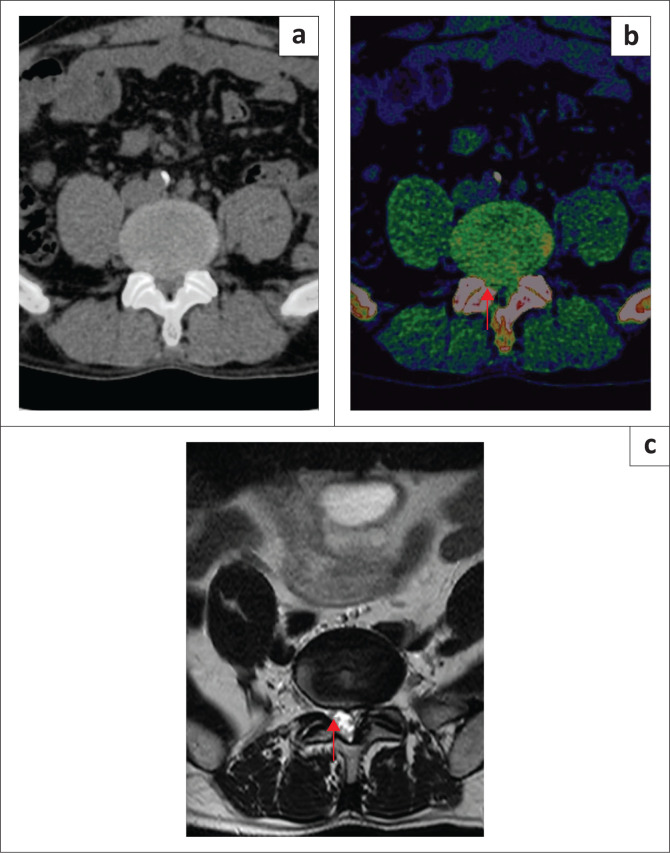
A 58-year-old man who presented with lower back pain and paraesthesia in the right lower limb: (a) Bulging L5/S1 intervertebral disc with impingement of the traversing right S1 nerve root was underestimated on standard grayscale axial CT. (b) Diagnosis of spinal nerve root impingement was made by all readers on the axial colour-coded electron-density reconstruction images. (c) Spinal nerve root impingement was confirmed on axial T2-weighted MRI (red arrow).
